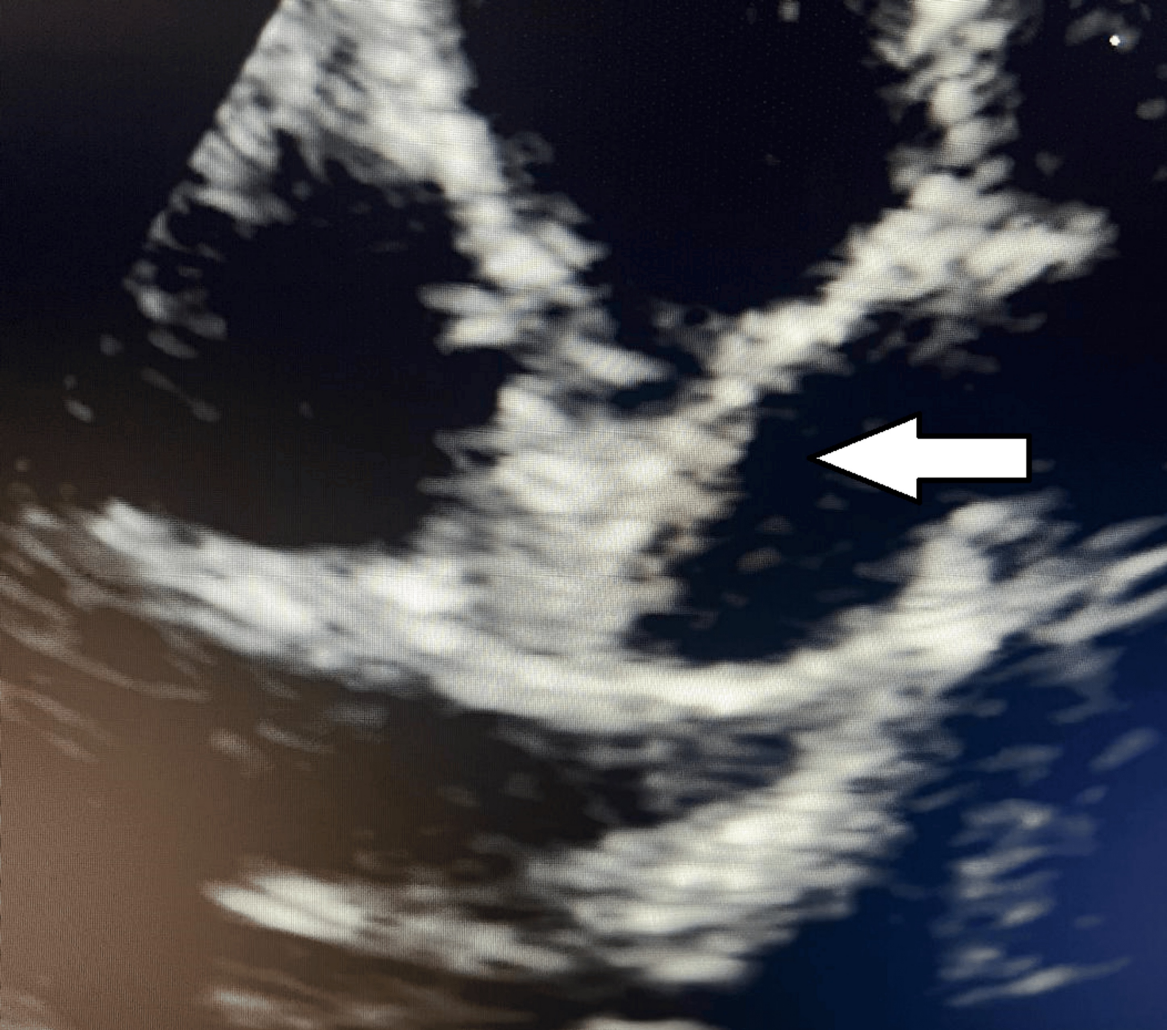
Follow-up echocardiography after anticoagulation therapy This follow-up image shows the previously dilated left anterior descending (LAD) artery without visible thrombus, indicating successful resolution of the thrombus following anticoagulation therapy.
